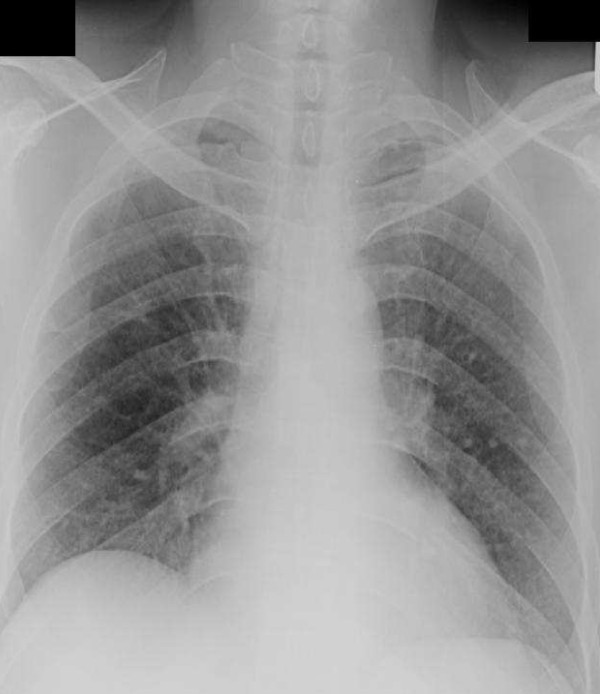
Chest radiograph showing interstitial infiltration in both lower lung fields.
